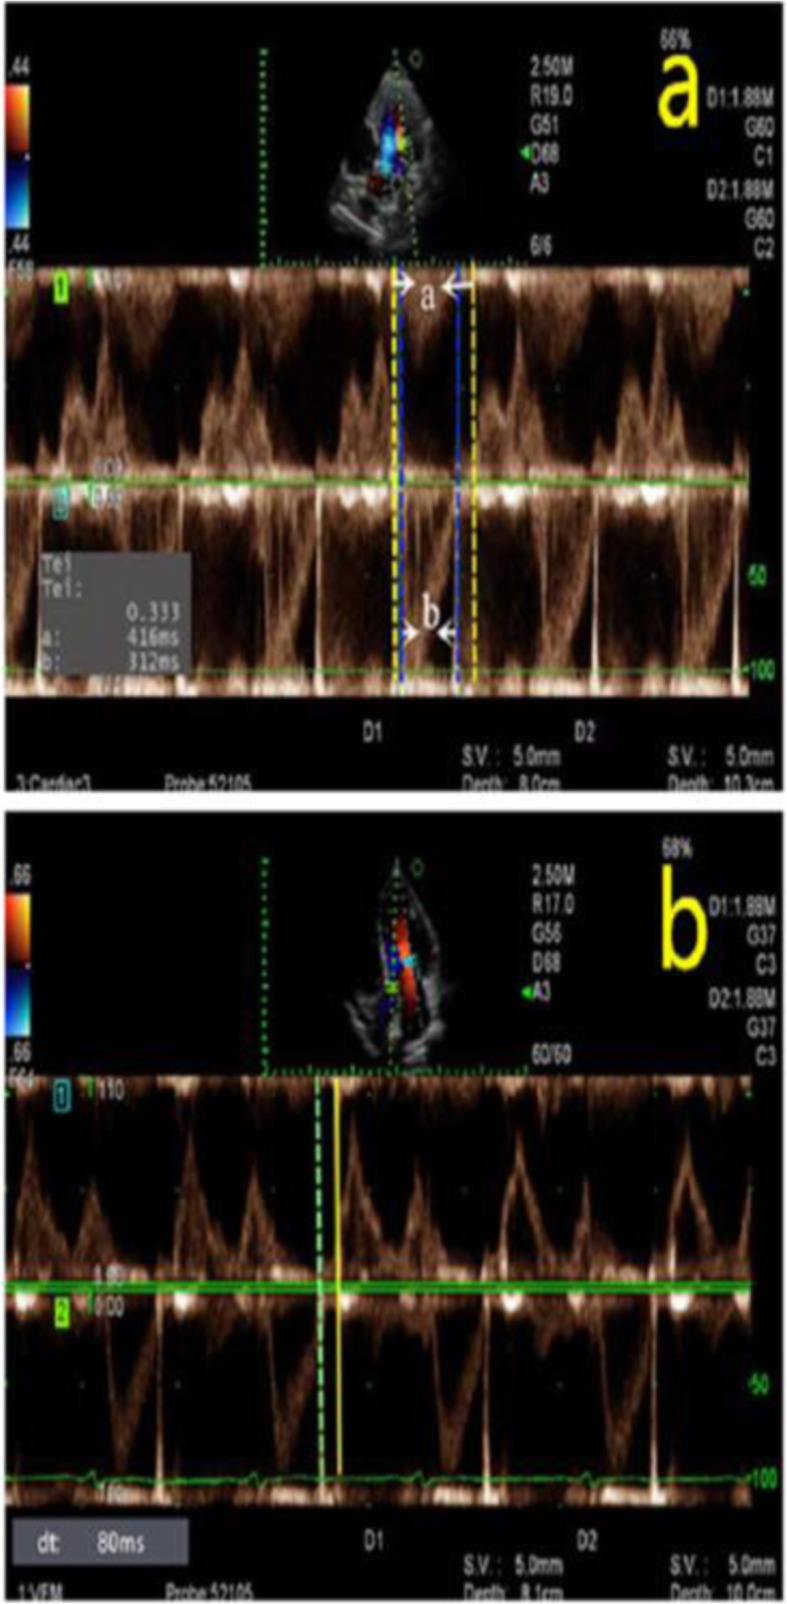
a. Tei index is defined by the equation (a-b)/b, where “a” represents the interval between cessation and onset of mitral inflow, and “b” represents the ejection time of the left ventricular outflow. b. The isovolumic relaxation time was measured by dual Doppler echocardiography, with the green imaginary line representing the end of aortic flow and the yellow full line representing the beginning of mitral flow
